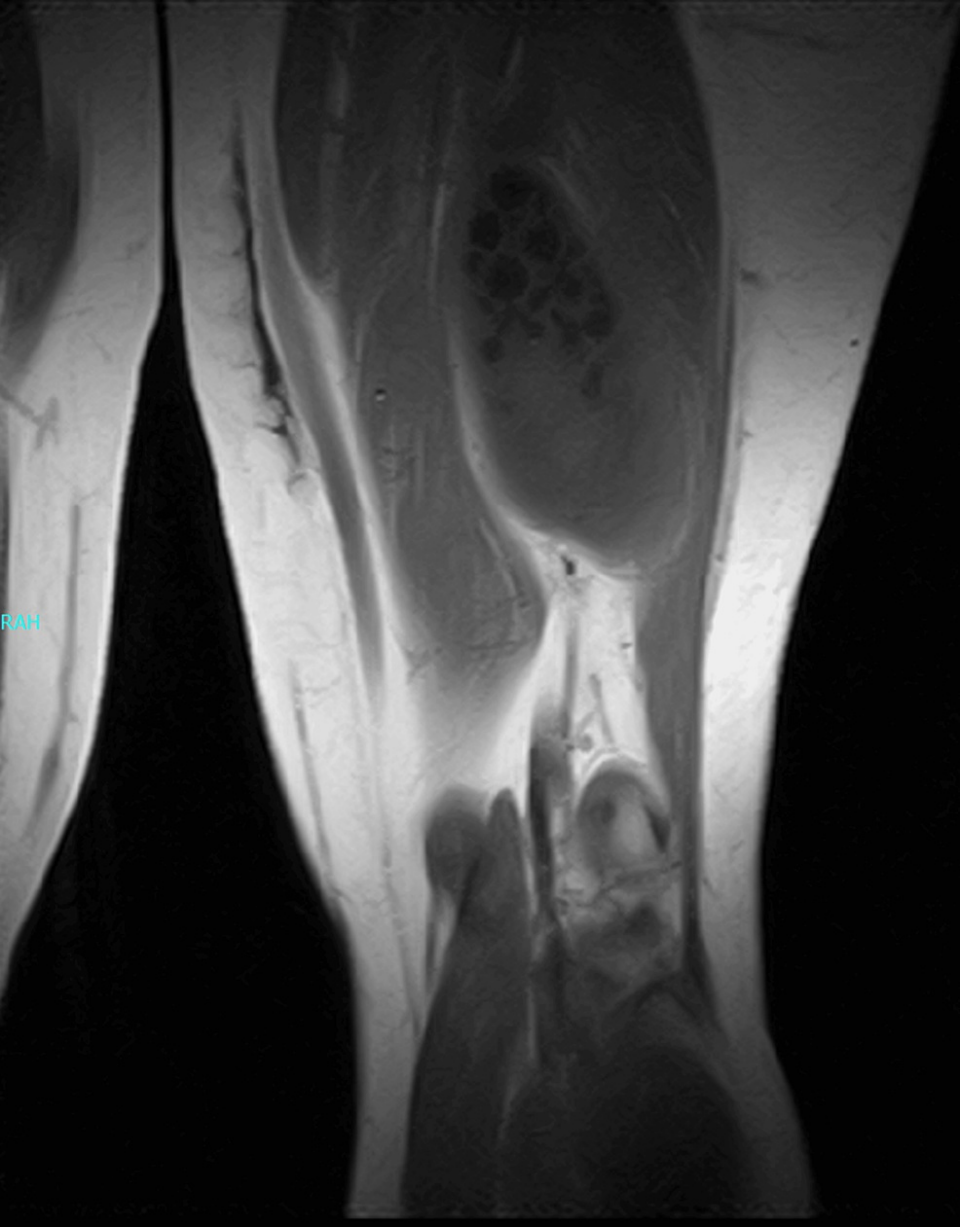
MRI of the lesion in the thigh (before surgery)
